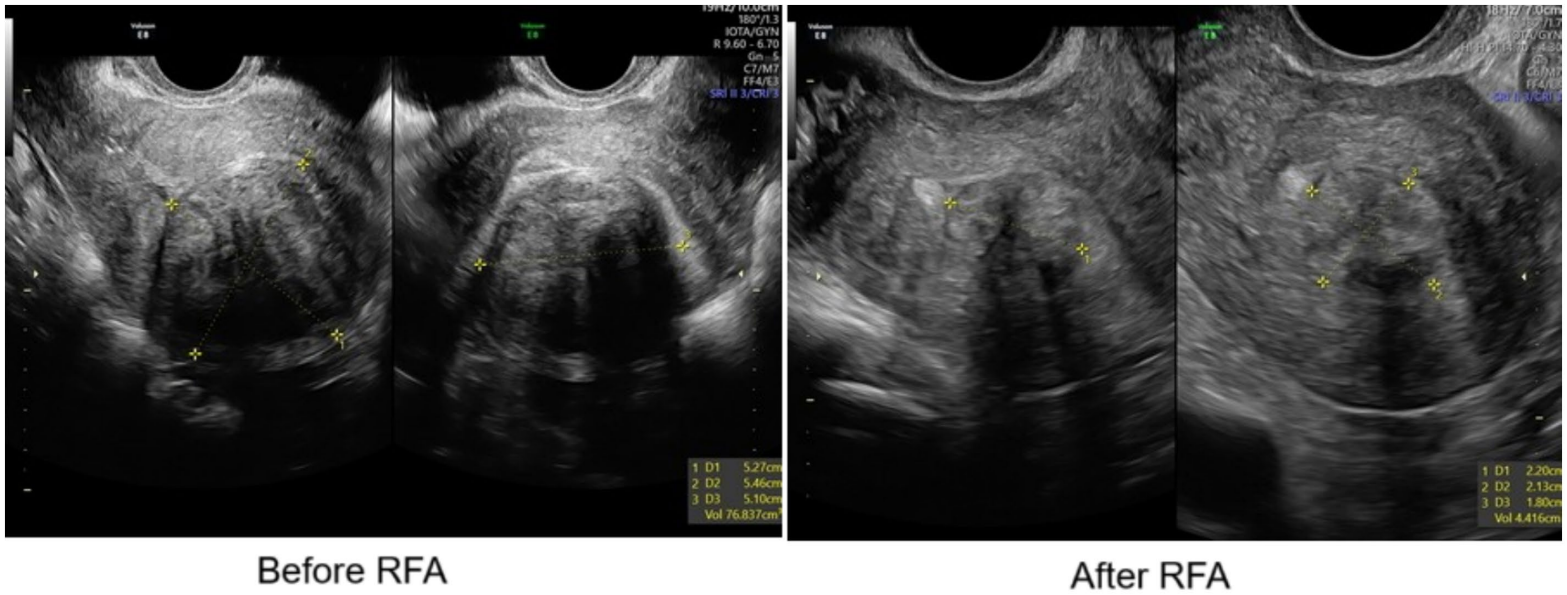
Ultrasound before RFA (left) and after RFA (right).
